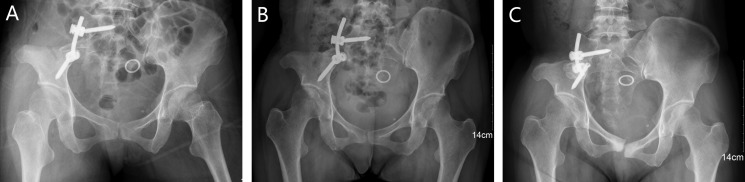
A 37-year-old female (case 26) with diagnosis of pelvis chondrosarcoma affecting zone I (A) AP radiograph of the pelvis after bone tumor resection and reconstruction with an autograft bone and screw-rod system. (B) AP radiograph showing the implant loosening 3 months post-operatively without functional impairment. (C) at 51.3 months post-operatively, AP radiograph showing failure of the implant, pseudoarthrosis formation was found in the resection site, and degeneration was developed in the symphysis pubis.
